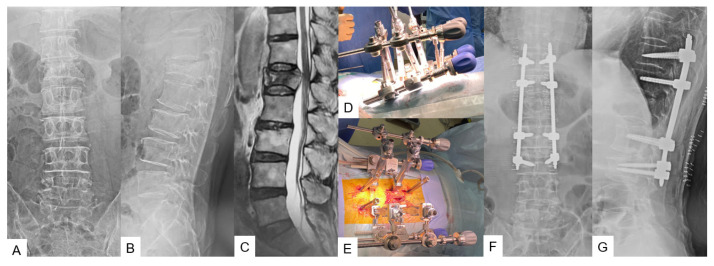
Case 3, 73-year-old male, L1 osteoporotic vertebral fracture, T11-L3 percutaneous posterior reduction. (A) Preoperative anteroposterior radiogram, (B) Preoperative lateral radiogram, (C) Preoperative T2 weighted MR imaging, (D,E) Intraoperative image, (F) Preoperative anteroposterior radiogram, (G) Postoperative lateral radiogram.
